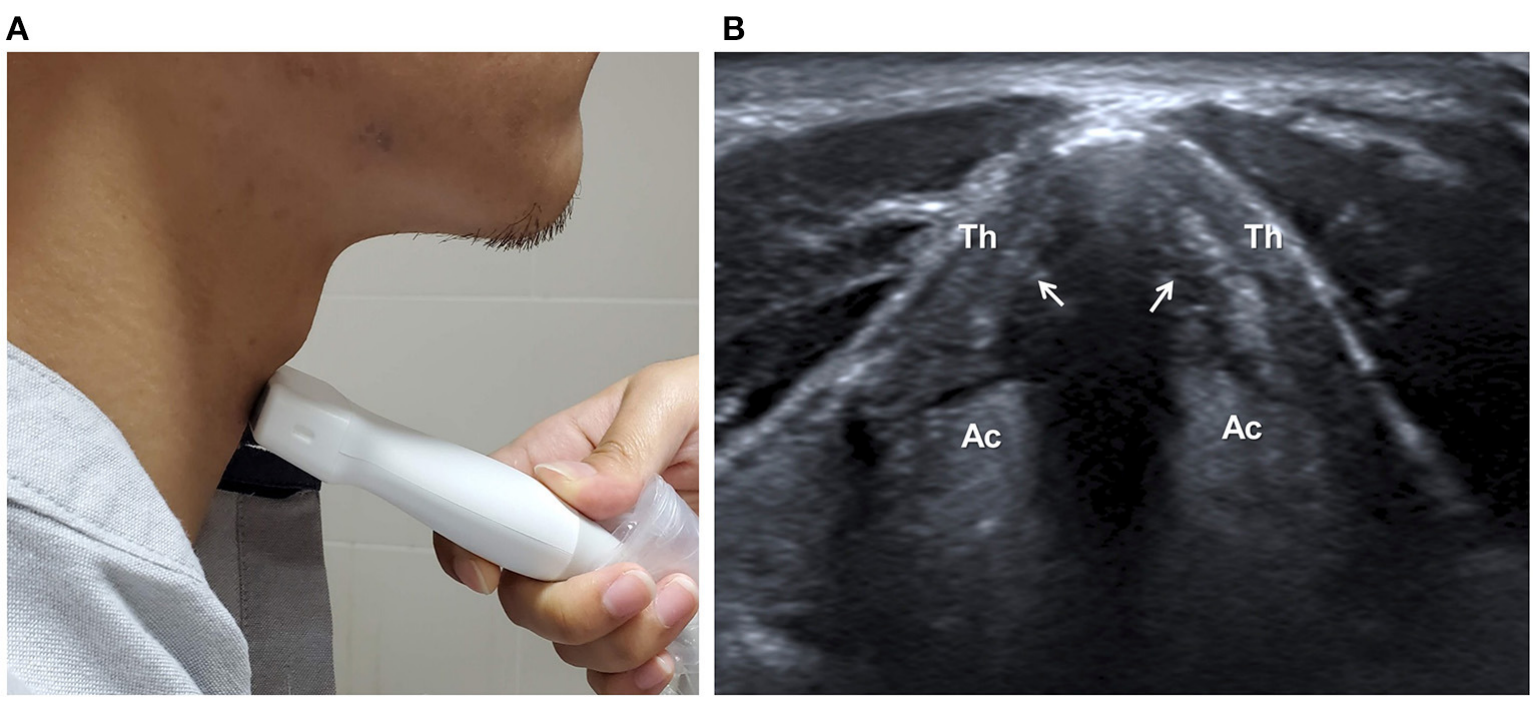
Ultrasonographic assessment of vocal cords. The transducer is placed anterior to the thyroid cartilage (Th) in transverse plane (A). The structure and movement of vocal cords (arrows) can be observed (B). Ac, Arytenoid cartilage.
